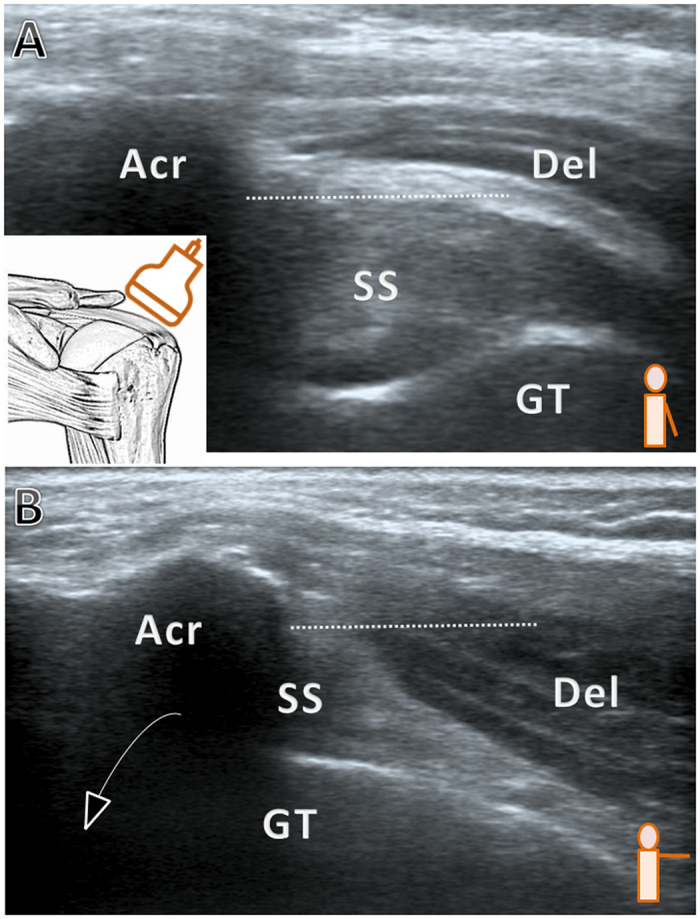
Grade 0, no impingement (A) The arm is rested beside the trunk (B) The arm is elevated to 90 degrees in the horizontal plane. The sonographic presentation of grade 1 impingement is the same as that of grade 0 but with pain during shoulder elevation. Acr, acromion; Del, deltoid; GT, greater tuberosity; SS, supraspinatus tendon; dashed line: the inferior edge of the acromion.
